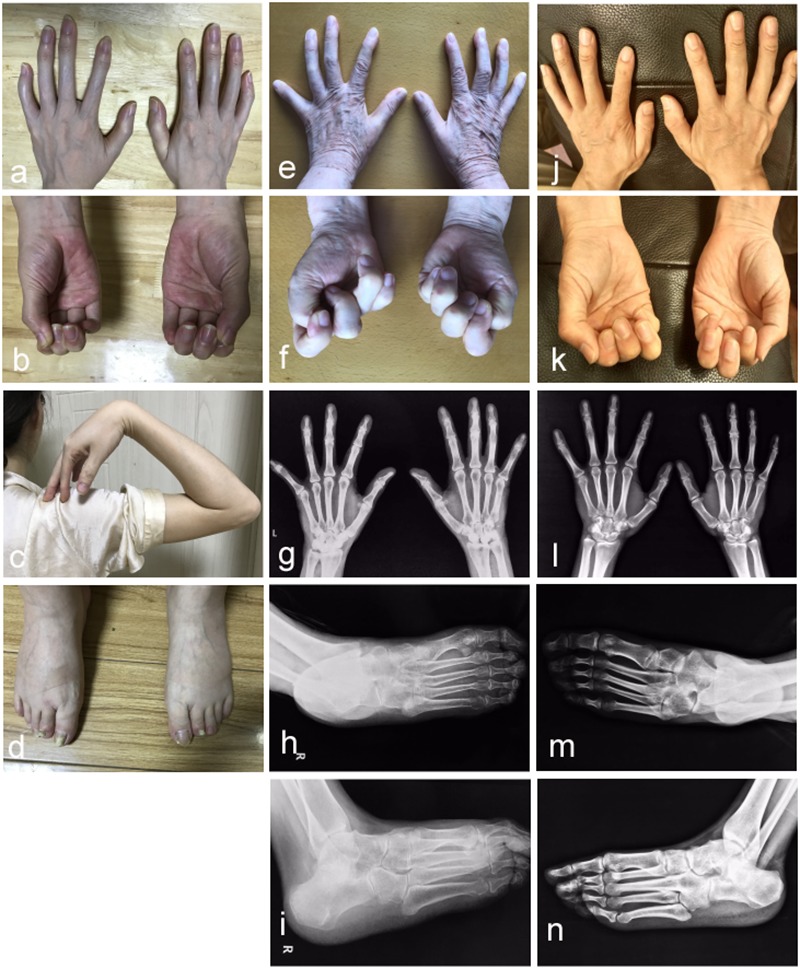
Photographic features of hands and feet in the affected individuals. Abnormal joint osseous fusion in fingers and difficulties in making fists were seen in all of patients II-4 (a,b), I-2 (e,f) and II-1 (j,k). For patient II-4, the motion of the elbow joint is normal (c), and a little short phalange of second toe is noticed in both her feet (d). Radiographs of the hands reveal that patient I-2 (g) and II-1 (l) have proximal symphalangism in hands. Radiographs of right feet in anteroposterior and oblique view show that patient I-2 (h,i) presents proximal symphalangism in toes and tarsal coalitions, while patient II-1 (m,n) has both proximal and distal symphalangism in toes and tarsal coalition.
